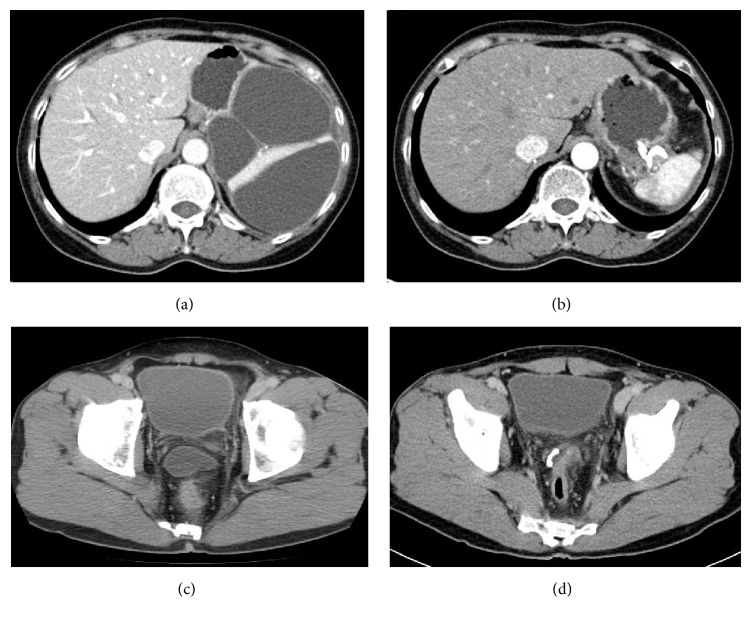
Computed tomography images (a–d). (a) A 13 cm pseudocyst in a patient with IgG4-related pancreatitis, and (b) resolution image of the pseudocyst after stent placement. (c) A 5.5 cm perirectal abscess in a patient with previous perforated diverticulitis, and (d) reduction in abscess size after stent placement.
